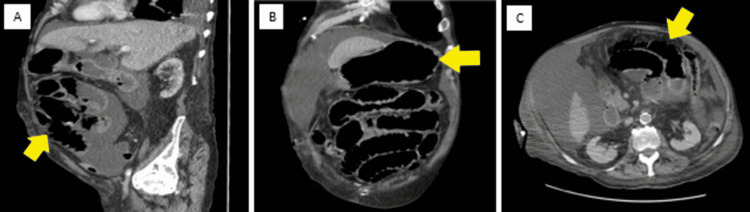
A) sagittal, B) coronal, and C) axial: presence of small bowel obstruction on abdominal/pelvis CT.
